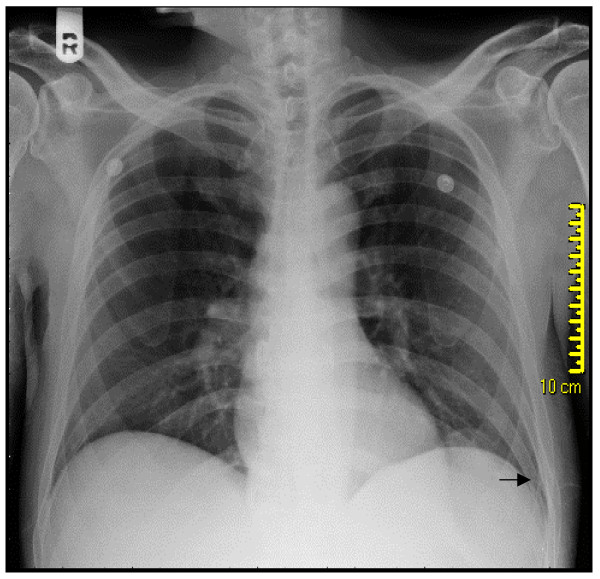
Initial chest X-ray: fracture of left 8th, 9th and 10th ribs (arrow).
